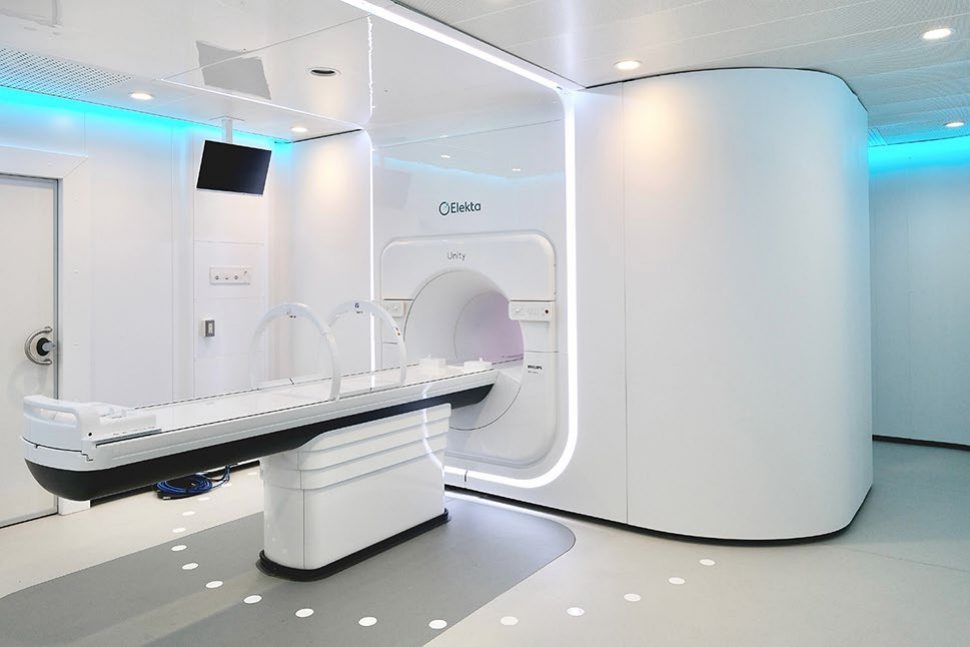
Elekta Unity 1.5-T MR-Linac system. The internal structure consists of a linear accelerator rotating gantry placed outside of the MRI magnets
